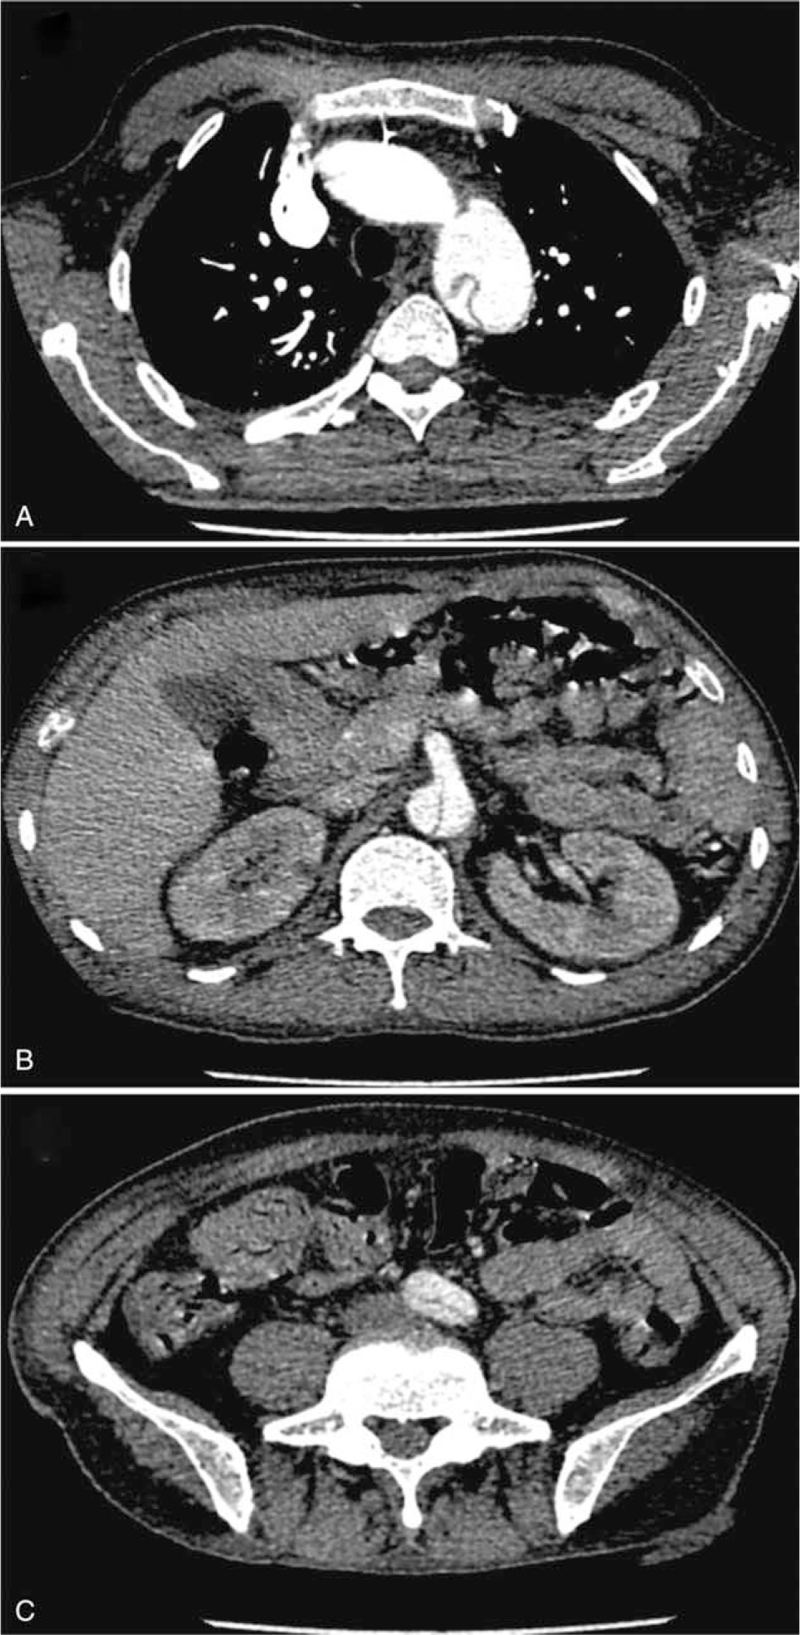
The Chest and abdomen CT angiography (CTA) results of the patient. The CTA showed a Stanford type B aortic dissection, with an incision in the descending thoracic aorta, involving the thoracic aorta (A), abdominal aorta (B), and bilateral common iliac arteries (C).
